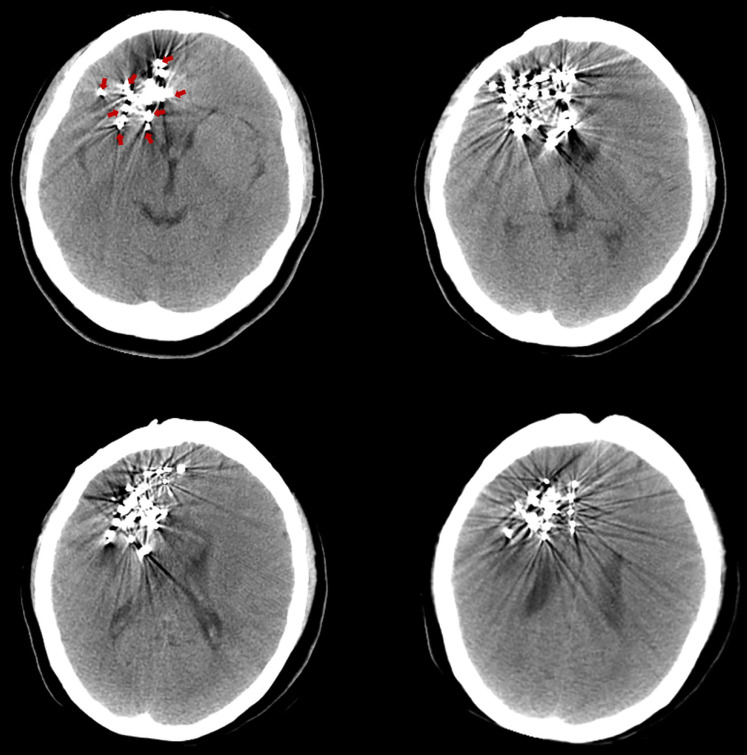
Three days after the ^125^I implantation, a CT scan was carried out to exclude delayed damage to the brain. The images show different slices of the brain with ^125^I seeds. (Arrows indicate the ^125^I seeds implanted in the brain).
